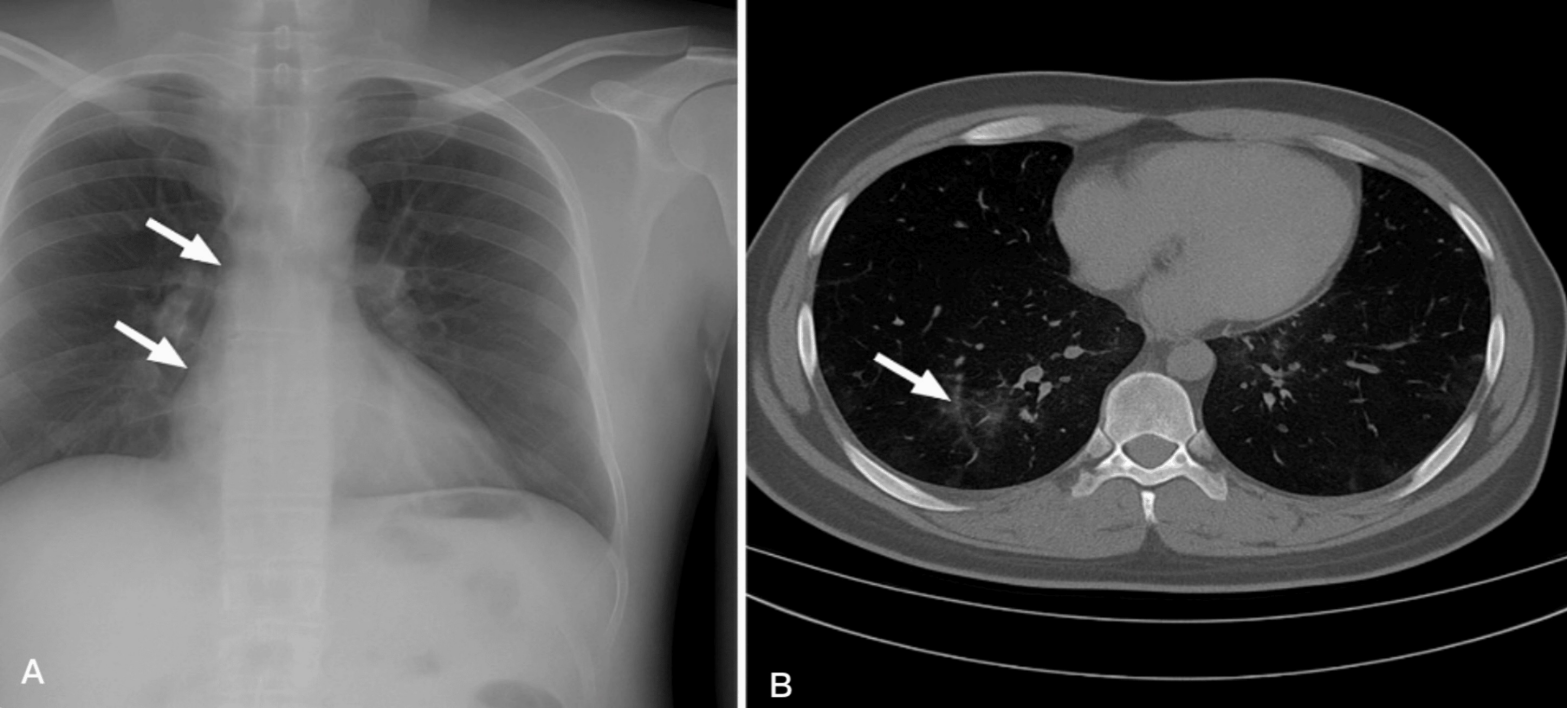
Radiographic and High-Resolution Computed Tomography (HRCT) Findings in a Postoperative ICU Patient With MDR Acinetobacter baumannii Pneumonia (A) Frontal chest radiograph shows ill-defined opacities in the right lower and mid-lung zones (white arrows), suggestive of localized consolidation. (B) Axial CT scan of the chest confirms patchy consolidation with surrounding ground-glass opacities in the right lower lobe (white arrow), without pleural effusion. These findings are consistent with a focal atypical pneumonia, later confirmed microbiologically as multidrug-resistant (MDR) *Acinetobacter baumannii* infection in a post-surgical intensive care unit (ICU) patient
